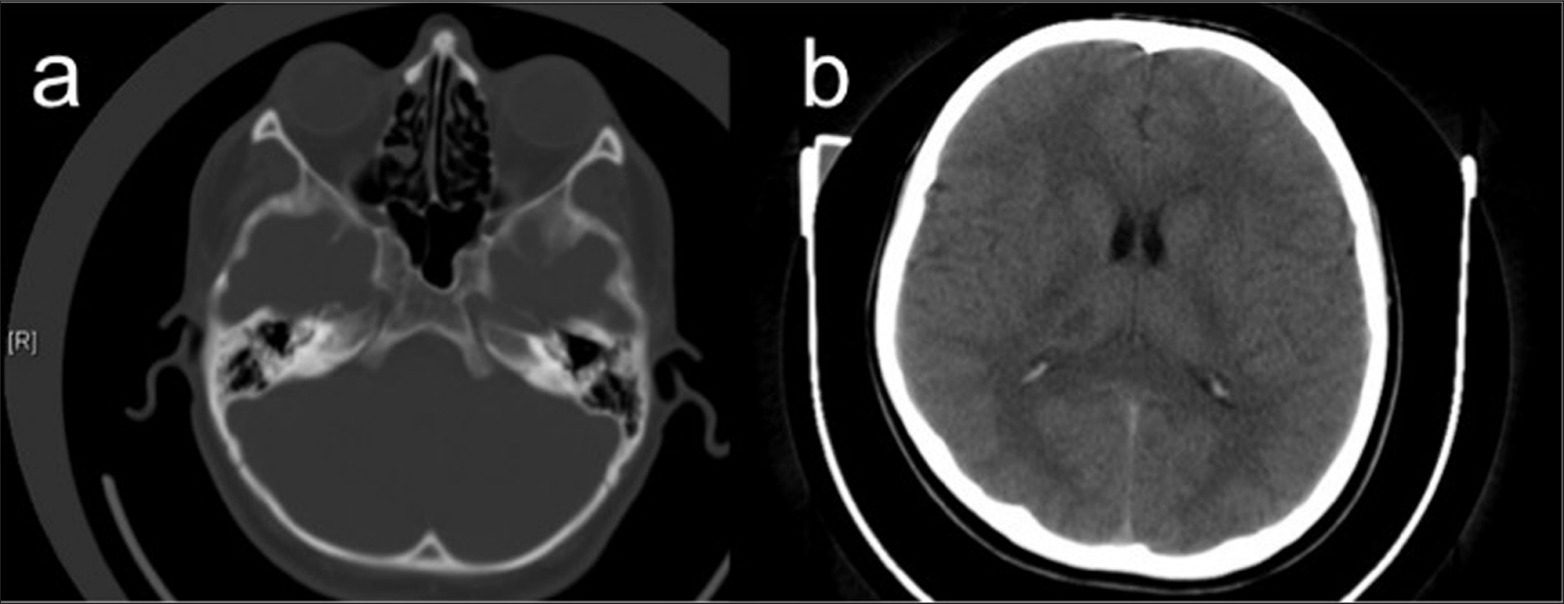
An axial CT image at bone window reveals absent right bony carotid canal (a); cranial CT image reveals hypodense lesion at right subthalamic region about 1 cm in diameter (b) in Case 2.
